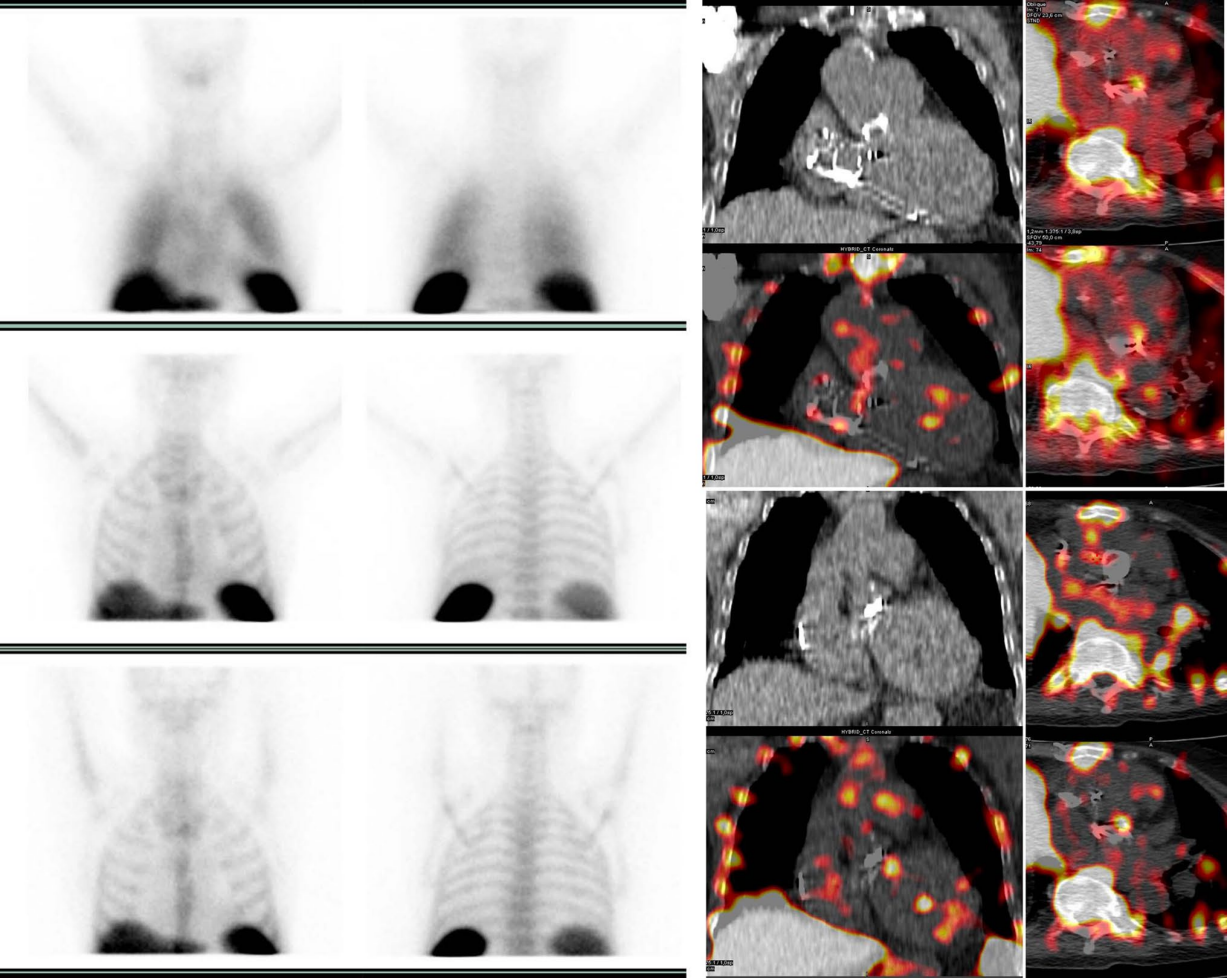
Radiolabeled leukocyte (^99m^Tc-HMPAO WBC) in a patient with suspected IE bearing aortic and mitral prosthetic valve and CIED. Planar images (right panel top-down at 30 min, 6 h, and 20 h) after the injection of the radiopharmaceutical and SPECT/CT (middle column coronal CT and superimposed SPECT/CT, left column transaxial superimposed SPECT/CT at different levels) showing pathological uptake on the lateral and medial sides of the mitral valve prosthesis (images were acquired on Discovery-670, GE Healthcare). No signs of infection are present along the CIED
